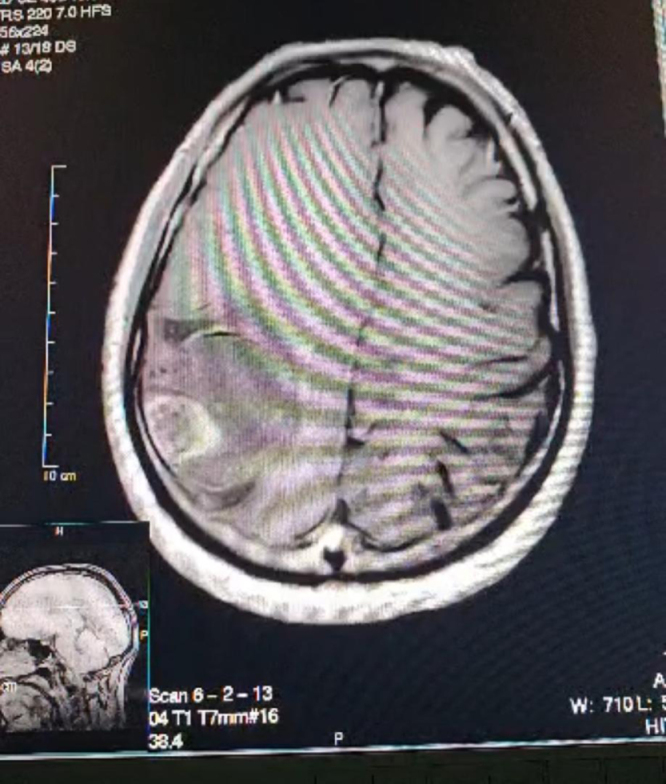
Computed tomography (CT) scan of the brain showing a well-circumscribed, hyperdense parenchymal lesion.
